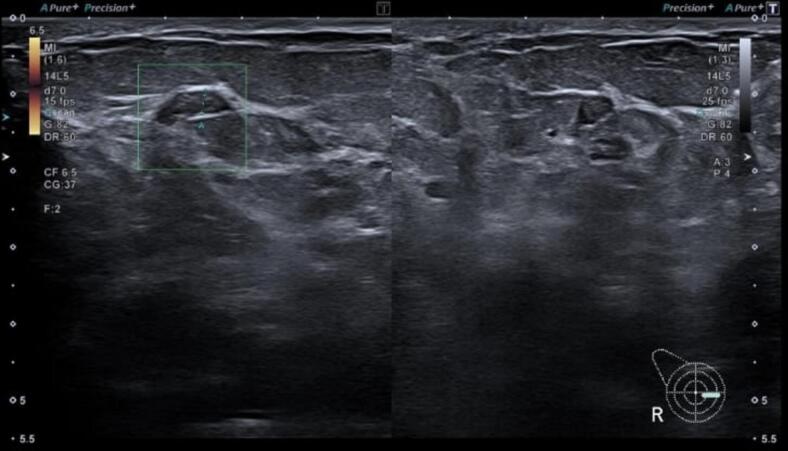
Ultrasound of right breast after conservative treatment showed improvement.
